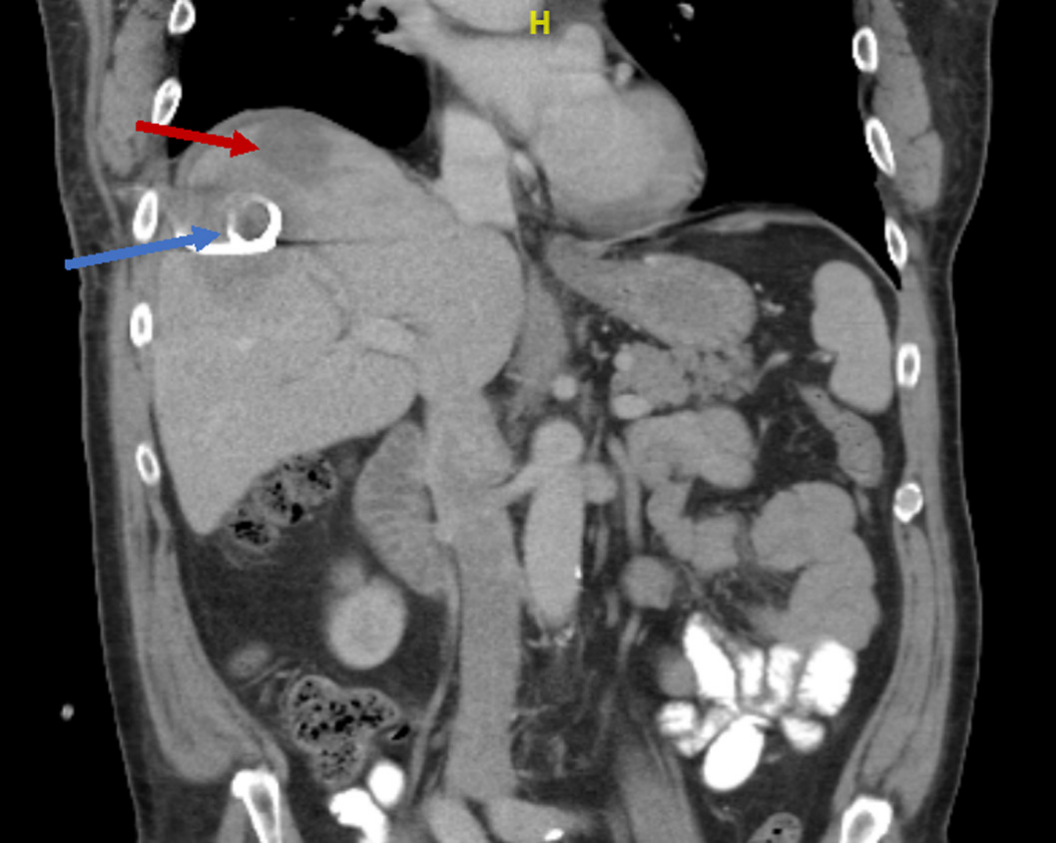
CT abdomen and pelvis with contrast from the patient's fifth episode of recurrent liver abscess. This shows a large abscess (red arrow) within the liver dome, measuring 8.2 cm. There is a heterogeneous abnormal signal within the right hepatic lobe inferior to the liver dome abscess, corresponding to the previously drained abscess cavity; the percutaneous drainage catheter is noted within this region (blue arrow).
